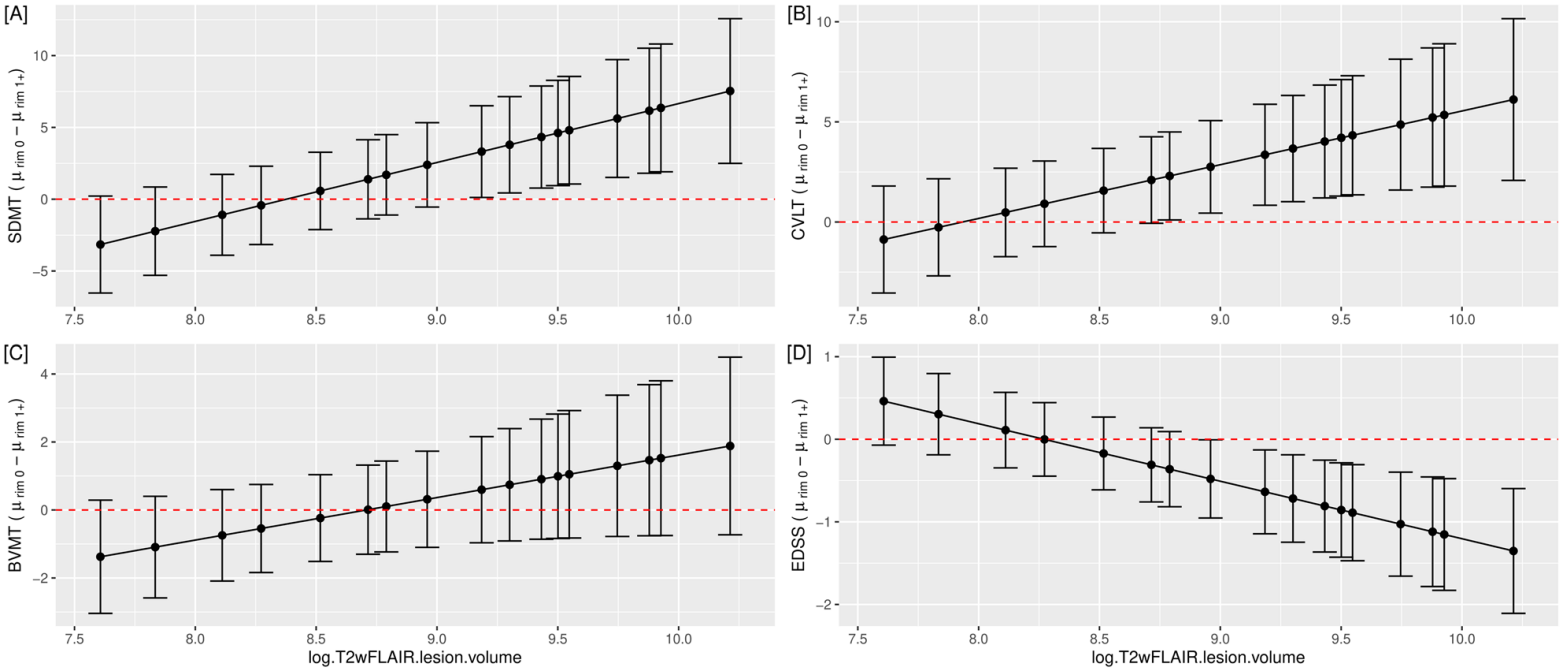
Association of rim+ lesions with disability measures after accounting for clinical and imaging covariates. Patients with both higher total lesion volume on FLAIR and at least one rim+ lesion performed worse on SDMT (A), CVLT-II (B), and EDSS (D) as demonstrated by 95% confidence intervals of the difference of mean disability scores between patients with zero rim+ lesions minus patients with at least one rim+ lesion. The 95% confidence intervals of the means are model-based estimates. The final model included current treatment duration, age, gender, total lesion volume on FLAIR, and the number of rim+ lesions (statistically significant covariates). Performance on BVMT-R (C) did not reach clinical significance in the model.
